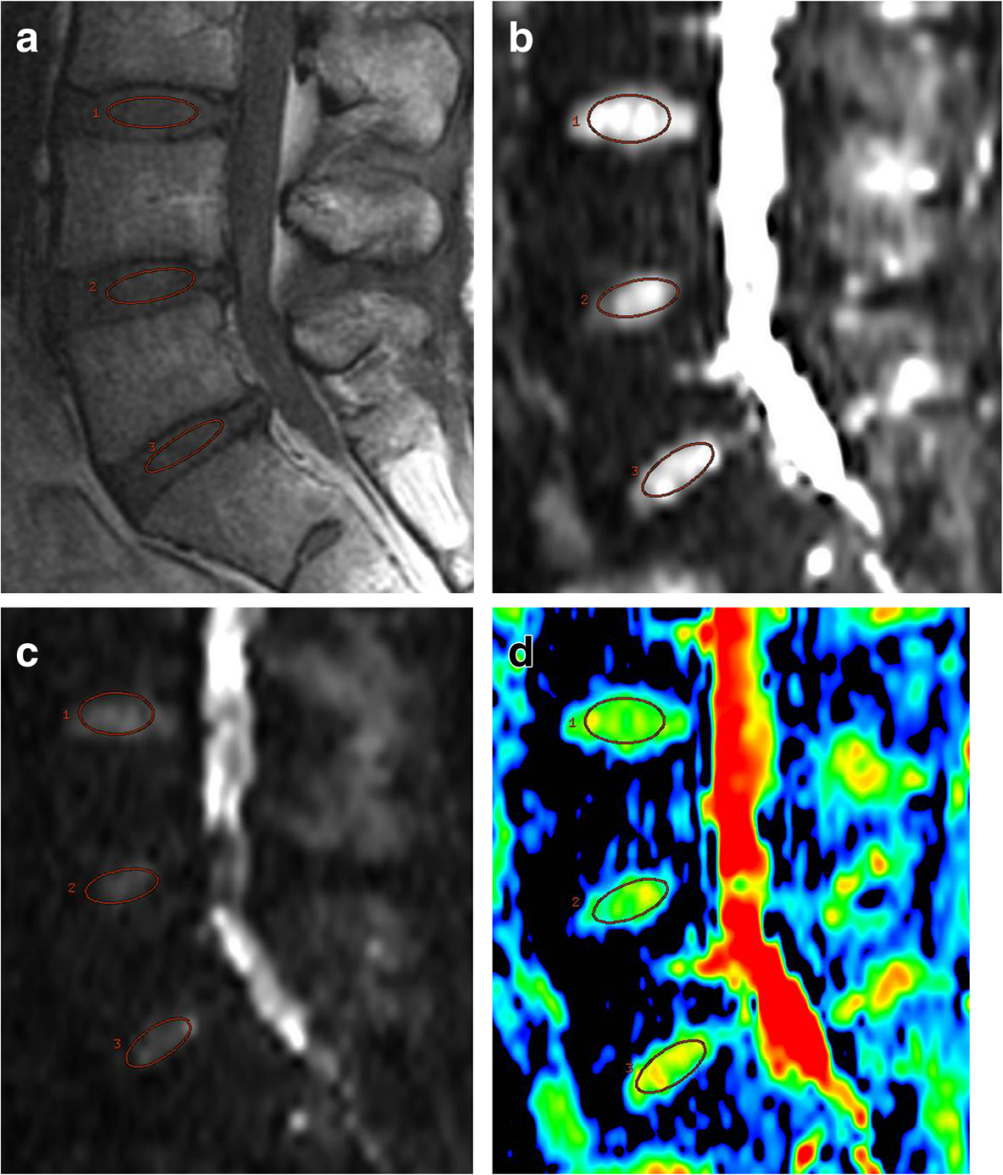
Measurement of the apparent diffusion coefficient (ADC) in lumbar degenerated discs. Mid-sagittal T2-weighted images were chosen and a region of interest (ROI, 50 mm^2^) placed in the central half of each disc. Sagittal fast spin echo (FSE) T2-weighted images (a) and diffusion-weighted images (DWI) with *b* factors of 0 (b) and 500 s/mm^2^ (c). ROI in the T2-weighted image copied to the ADC map from the DWI at the same level (d)
